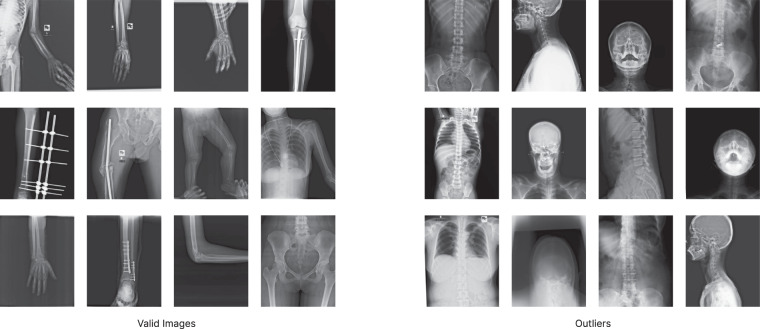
Example of valid (Left) vs outlier (Right) X-ray images. All the scans were manually filtered based on the parts of the body present in the scan, clarity of the scans and resolution. The scans containing only arm, shoulder, leg and hip regions were accepted.
